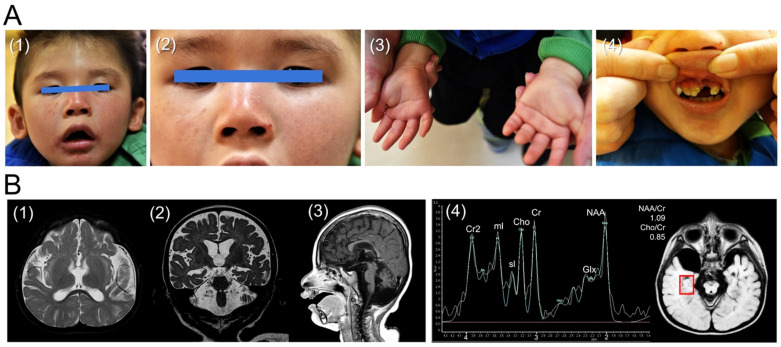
The photos and brain MRI of the proband. (A) Photos of the male proband at 3 years old. (1) General appearance of the face, which reveals a thin pointy nose and a mildly wizened face; (2) Zoom-in of the face shown in (1) showing sun-exposed skin photosensitivity as revealed by increased pigmentation over nose and cheek area, which is one of the typical characteristics of CS patients [1]; (3) bilateral single palmar crease; (4) severe dental caries. (B) Brain MRI of the proband performed at 2 years and 6 months old. (1) Axial short tau inversion recovery (STIR) images show generalized cerebral atrophy with ventricular dilatation, increased signal intensity in the subcortical white matter and external capsules. (2) Coronal T2-weighted images demonstrate hyperintensities in centrum semiovale and internal capsules. (3) Midline sagittal T1-weighted images reveal a thin corpus callosum and moderate atrophy of the brain stem and cerebellum, resulting in an enlarged cisterna magna. (4) MRS showing a decreased NAA/Cr ratio (1.09) in the gray matter of the medial temporal lobe. These combined neuroradiologic findings can assist in the diagnosis of CS.
